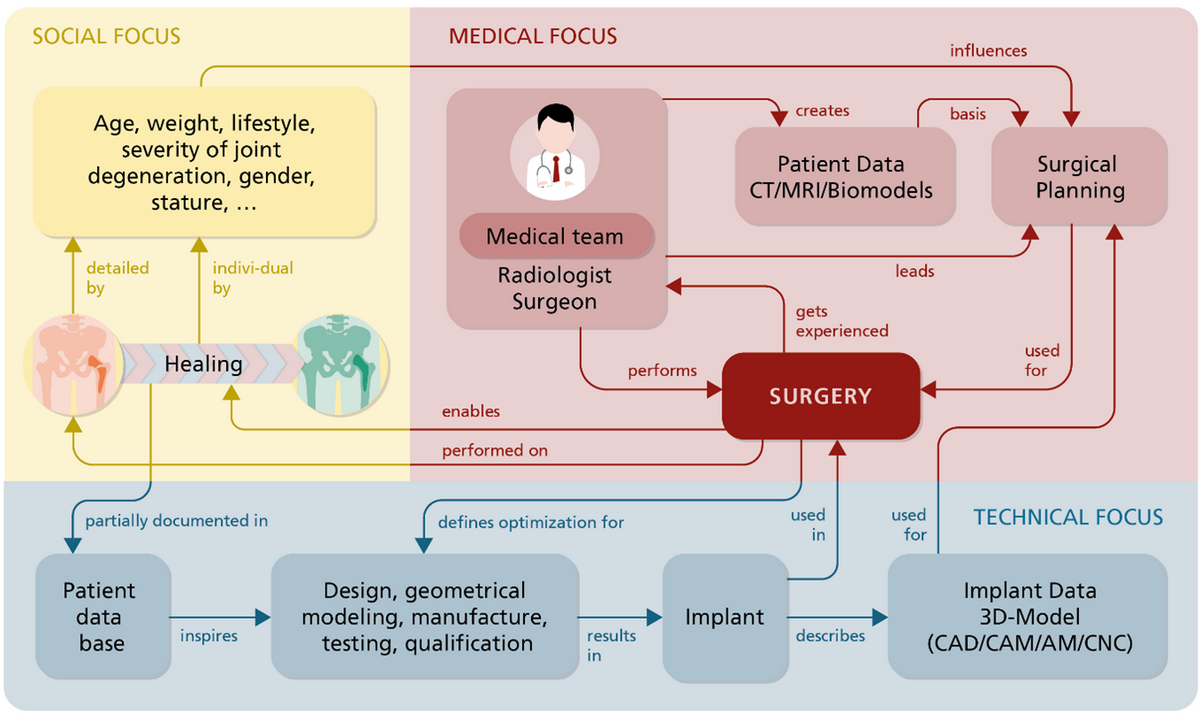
Incident-based networking of social aspects, medical factors, and technical aspects. AM: additive manufacturing; CAD: computer-aided design; CAM: computer-aided manufacturing; CNC: computer numerical control; CT: computed tomography; MRI: magnetic resonance imaging.
